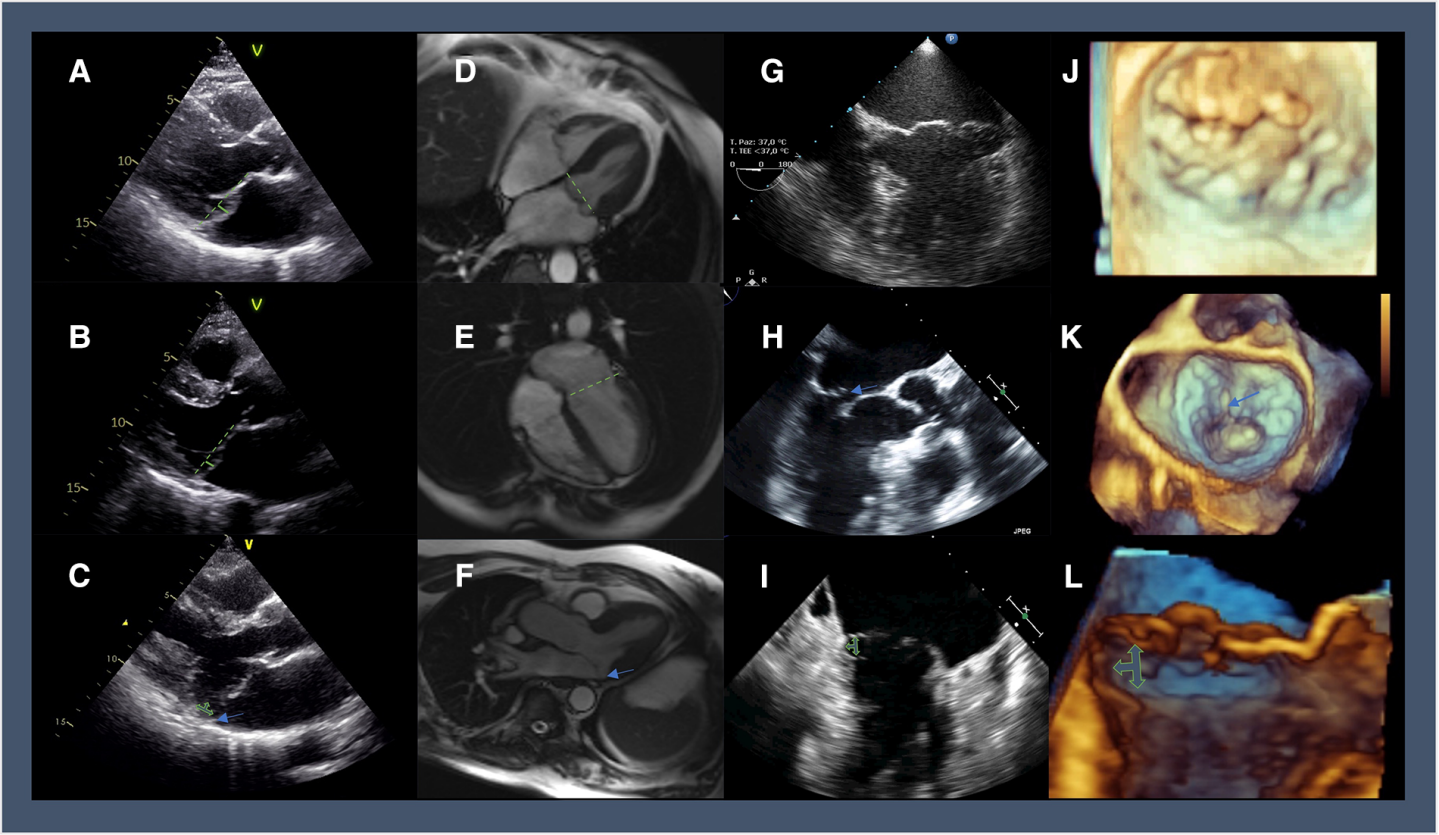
Multi-modality imaging highlighting the different features of MVP: 2D-TTE in parasternal long-axis view showing (A) diffuse thickening of the mitral tissue and the systolic displacement of posterior leaflets 3 mm beyond the plane of the annulus (green line); (B) leaflets thinning with a prolapse limited to posterior leaflets reaching cut-off of 2 mm (green line); (C) bileaflet prolapse and thickening of the mitral valve tissue with posterior MAD (green arrows; a blue arrow shows the detachment of postero-lateral annulus from posterolateral ventricular myocardium); Cine-CMR imaging in end-systolic 4-chamber view showing (D) Barlow's disease with bileaflet thickening of the mitral valve (green line representing the annulus plane); (E) fibroelastic deficiency with anterior leaflet thinning and prolapse in the left atrium; (F) MAD can be diagnosed in an SSFP three-chamber view in the systole (blue arrow); 2D-TEE (G) apical 4-chambers view showing Barlow's disease with a significantly enlarged mitral annulus and multisegmental prolapse and (H) 3-chamber of fibroelastic deficiency with P2 flail segment (blue arrow); (I) 2-chamber view of multiscallop prolapse with MAD (green arrow); 3D zoom aquisition of the mitral valve in surgical view showing Barlow's disease with multisegmental prolapse (J), and fibroelastic deficiency with P2 flail segment and ruptured chords (blue arrow) (K); cropped sagittal plane demonstrating a lateral view of the mitral valve with posterior MAD (green arrows) (L).
